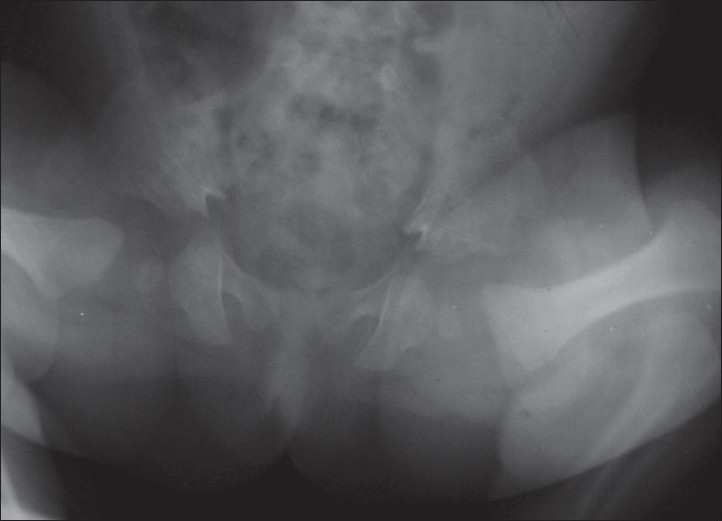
Radiograph showing bilateral short iliac wing and horizontal acetabulum with medial spurs
